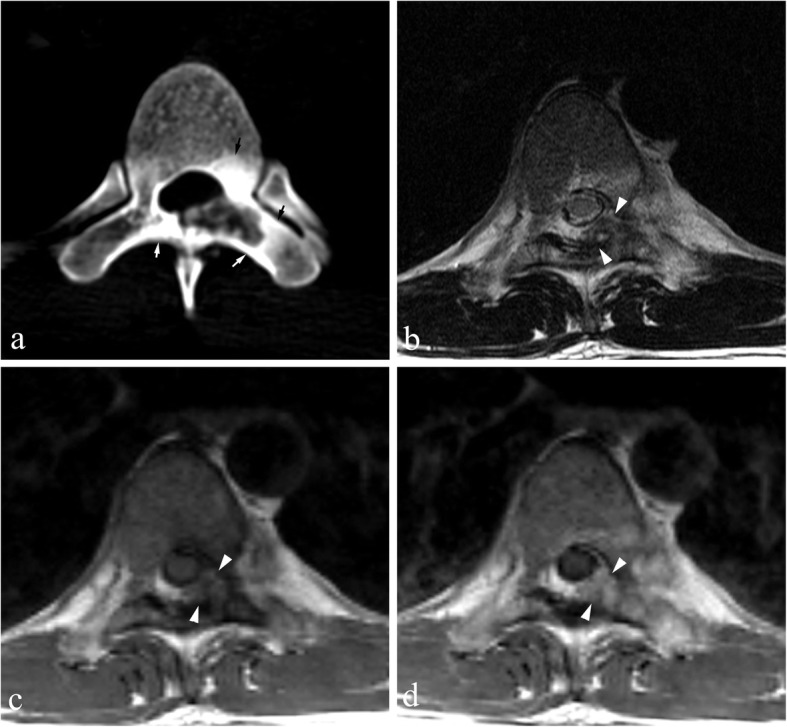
Osteoblastoma in a 25-year-old male, Enneking stage 3 on both CT and MRI. a and b Axial CT soft tissue and bone windows show a lesion causing extensive osteolytic destruction in L4 (which mainly affects the left pedicle, traverse process and lamina) with an incomplete sclerotic rim, unclear borders, and spotted calcifications. Note the thin flares in the epidural space (arrow). c and d Axial MRI T2WI and contrast-enhanced T1WI demonstrate the flare phenomenon adjacent to the tumor, i.e., abnormal swollen soft tissues in the spinal canal compressing the dural sac (arrow) lateral to the spinous process (arrowheads), showing hyperintensity on T2WI and marked enhancement with contrast
